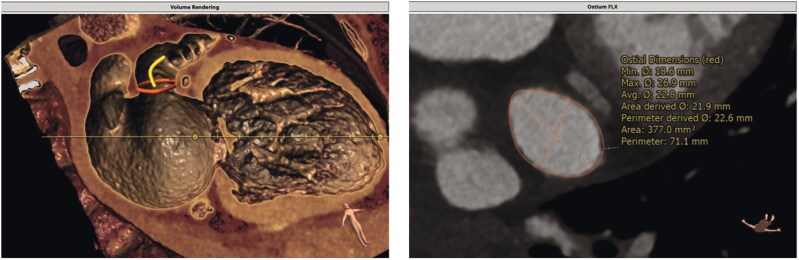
Pre-procedural planning with cardiac computed tomography to define the anatomy and size of the left atrial appendage.
